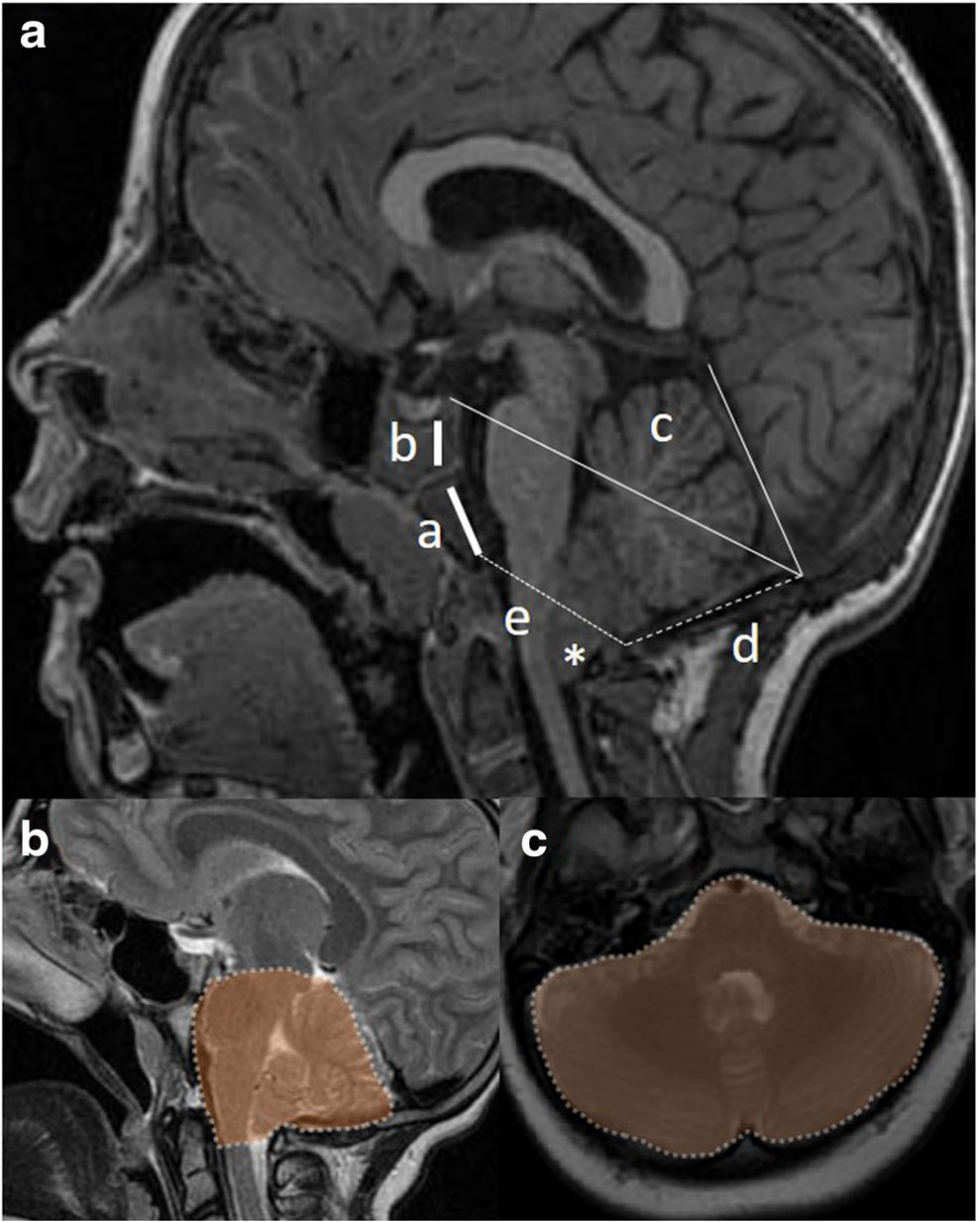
Main bi-dimensional PCF measurements on MRI (A): clivus basi-occiput (a), clivus basi-sphenoid (b), tentorial angle (c), supra-occiput (d), basion-opisthion line, and (e) to estimate the tonsillar descent (asterisk). Sagittal (B) and axial MRI (C) showing the template for the PCF three-dimensional assessment
